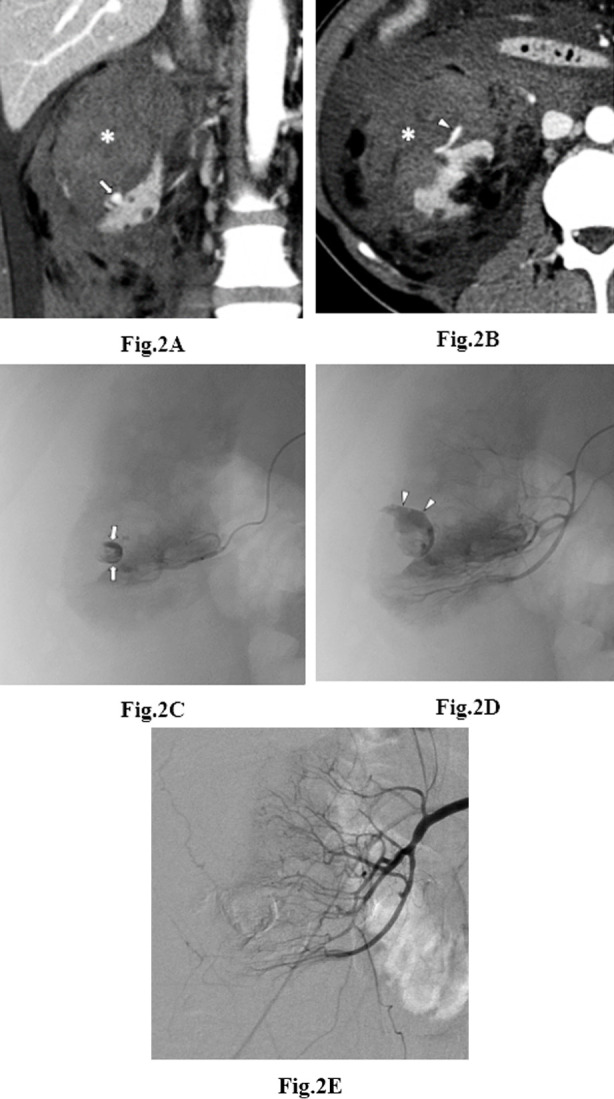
A 32-year-old woman (no. 8) with contrast extravasation. a, b Coronal (a) and axial (b) contrast-enhanced computed tomography scans show a nodular shape (arrow) and subsequent partial spread-out (arrowhead) of the contrast agent, suggesting hemorrhagic cyst rupture at the right renal cortex lower pole with a large subcapsular hematoma (asterisks). c, d Right renal selective angiograms showing contrast agent (arrows in c) confined to the renal cyst and contrast extravasation (arrowheads in d) beyond the cyst boundary. e Right renal angiogram after embolization with n-butyl cyanoacrylate showing partial embolization of the right renal artery.
